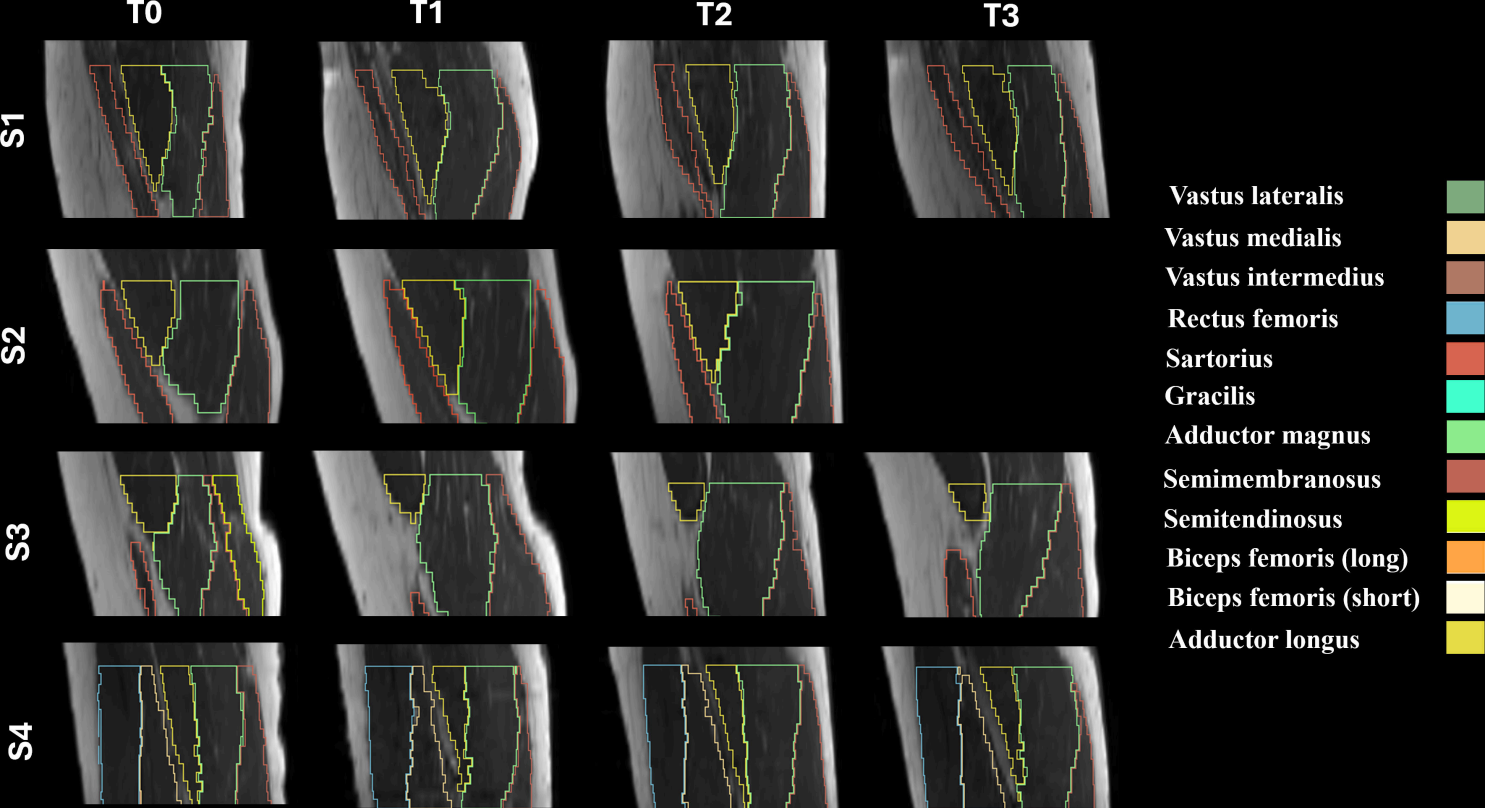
Extension of thigh muscle regions of interest across 4 participants (S1‐S4) in a representative sagittal plane for each time point (T_0_-T_3_). Each muscle is outlined with a distinct color. The regions of interest boundaries were consistently defined from the beginning of the semimembranosus to the last available slice of the rectus femoris, ensuring reproducibility across participants and time points. Magnetic resonance imaging scans covered a 30 cm range along the head-to-feet axis from the midpoint of the femoral head.
